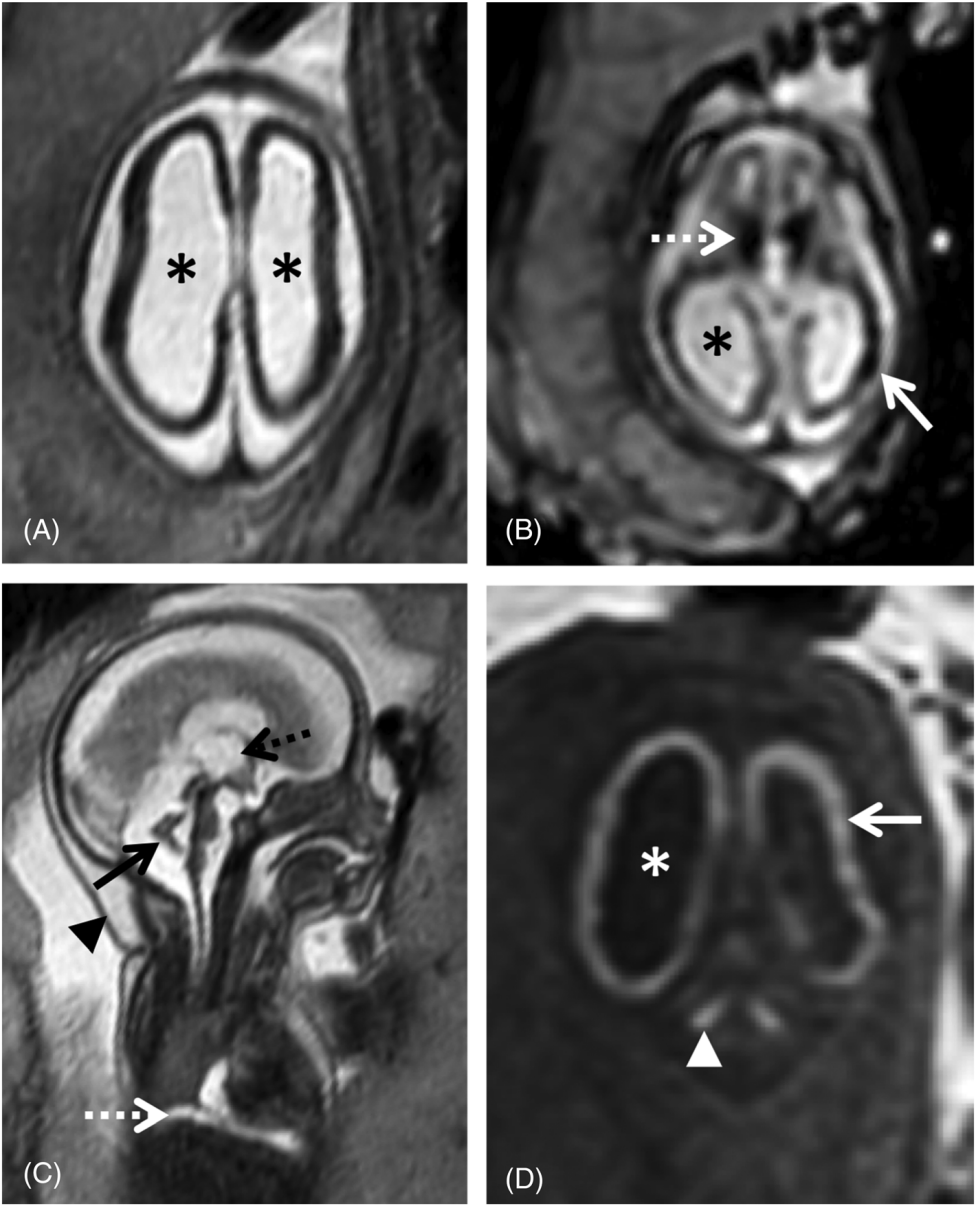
24 gestational weeks fetus referred for fetal magnetic resonance imaging (MRI) for suspected lissencephaly and cerebellar hypoplasia. T2w single shot fast spin echo axial (A) and sagittal (C), axial echo planar imaging (EPI) (B) and coronal T1WI (D) show a marked reduction of the cerebral parenchyma thickness, with diffuse low SI on T2WI (A) and severe ventriculomegaly (asterisk), including dilatation of the third ventricle (C, black dashed arrow). There is diffuse high SI on T1WI of the supratentorial parenchyma (D, white arrow) compatible with presence of calcifications, confirmed on EP images (B) and particularly evident in the basal ganglia (B, white dashed arrow). There is cerebellar hypoplasia (C, black arrow) associated with deep gray nuclei calcifications (D, white arrowhead). Furthermore, small pleural effusion can be seen on sagittal T2WI (C, white dashed arrow). Findings were confirmed on postmortem MRI (not shown). Additionally, there is skin edema/thickening of the skin (C, black arrow head)
